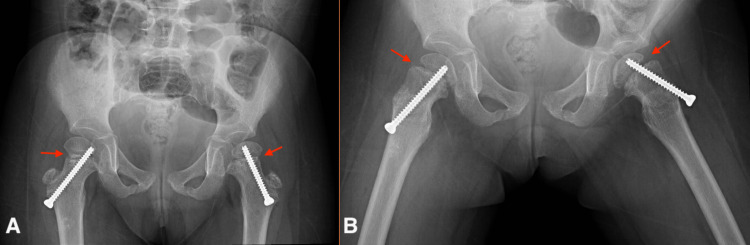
AP pelvis (A) and frog legs lateral (B) X-ray. Status post bilateral in situ pin fixation (red arrows).  No hardware complications.
